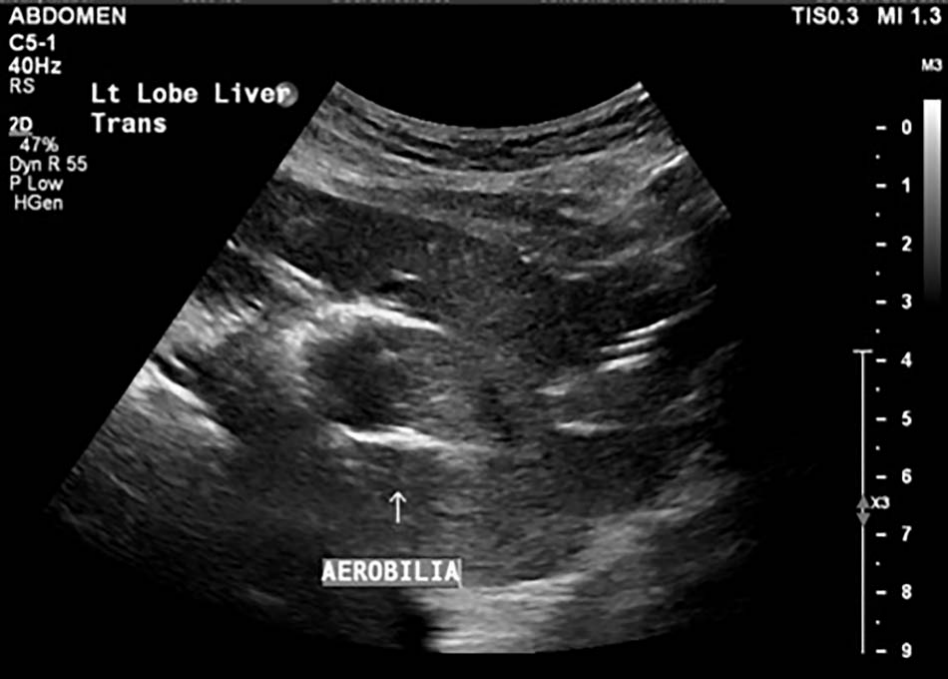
Abdominal ultrasound demonstrating pneumobilia.
